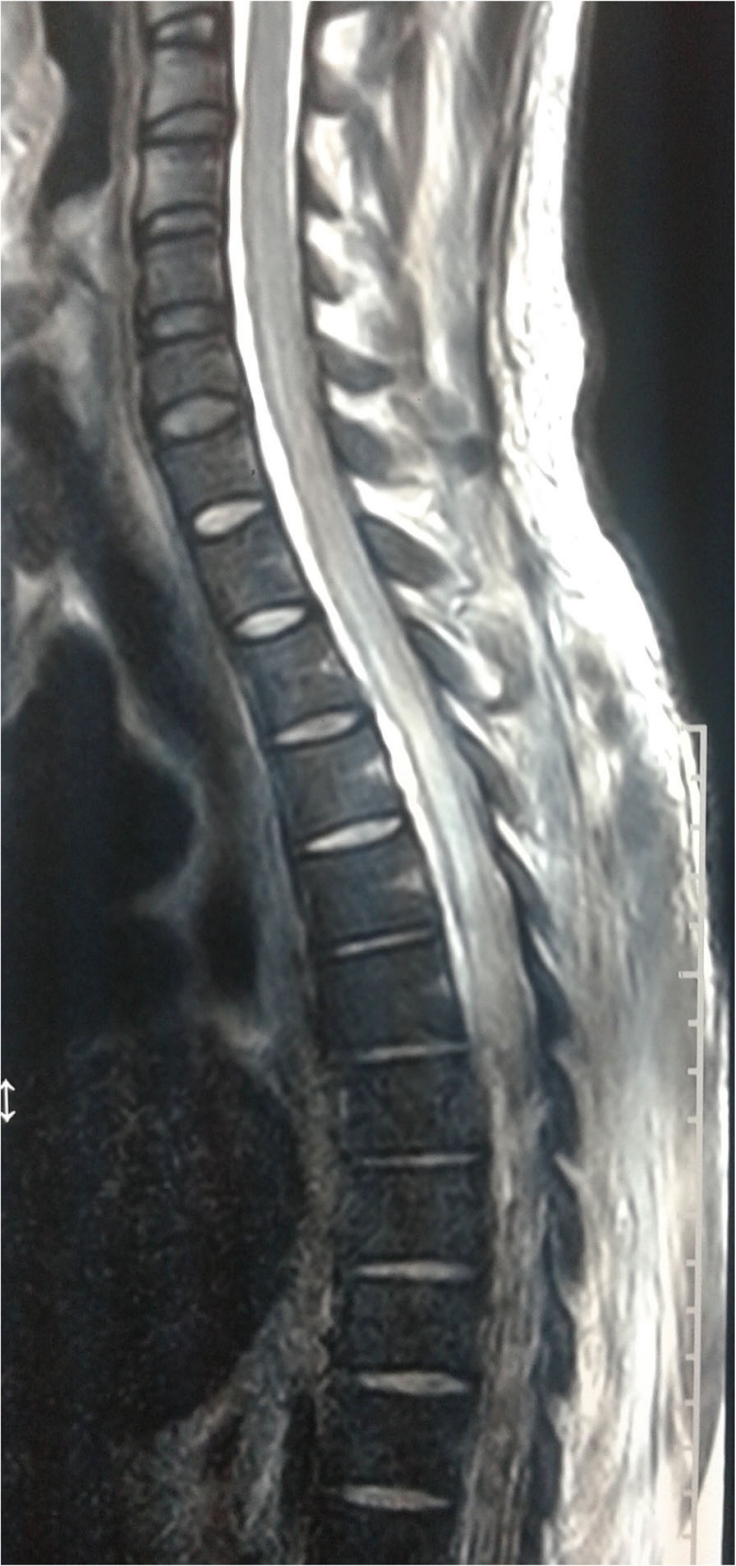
Magnetic resonance image of thoracic spine; sagittal view of thoracic segment of the spinal cord is shown. The image shows diffuse T2 high signal intensity within the spinal cord extending from second to 12th thoracic vertebral level
